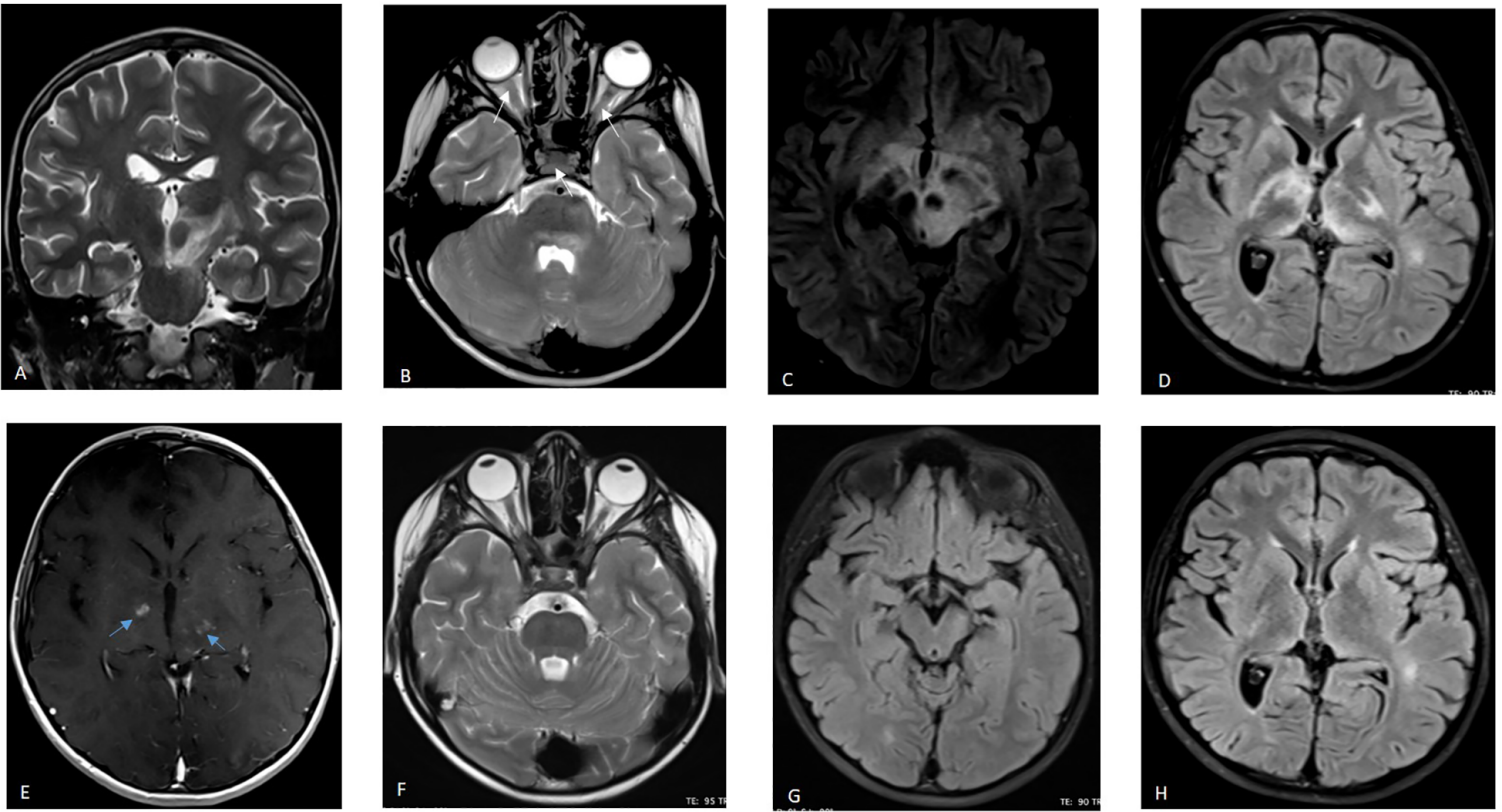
Brain MRI: coronal T2 weighted image (A), axial T2 weighted image (B) and axial FLAIR weighted images (C, D), show a poorly limited diencephalic infiltrative lesion extending into the brainstem, along with bilateral involvement of optic nerves and chiasm (white arrow). Axial T1-weighted image after gadolinium injection shows patchy bilateral contrast enhancement (blue arrows) in the basal ganglia (E). Follow-up brain MRI at eight months: axial T2 weighted (F) and FLAIR-weighted images (G, H) show marked reduction of the brainstem and diencephalic hypersignal.
